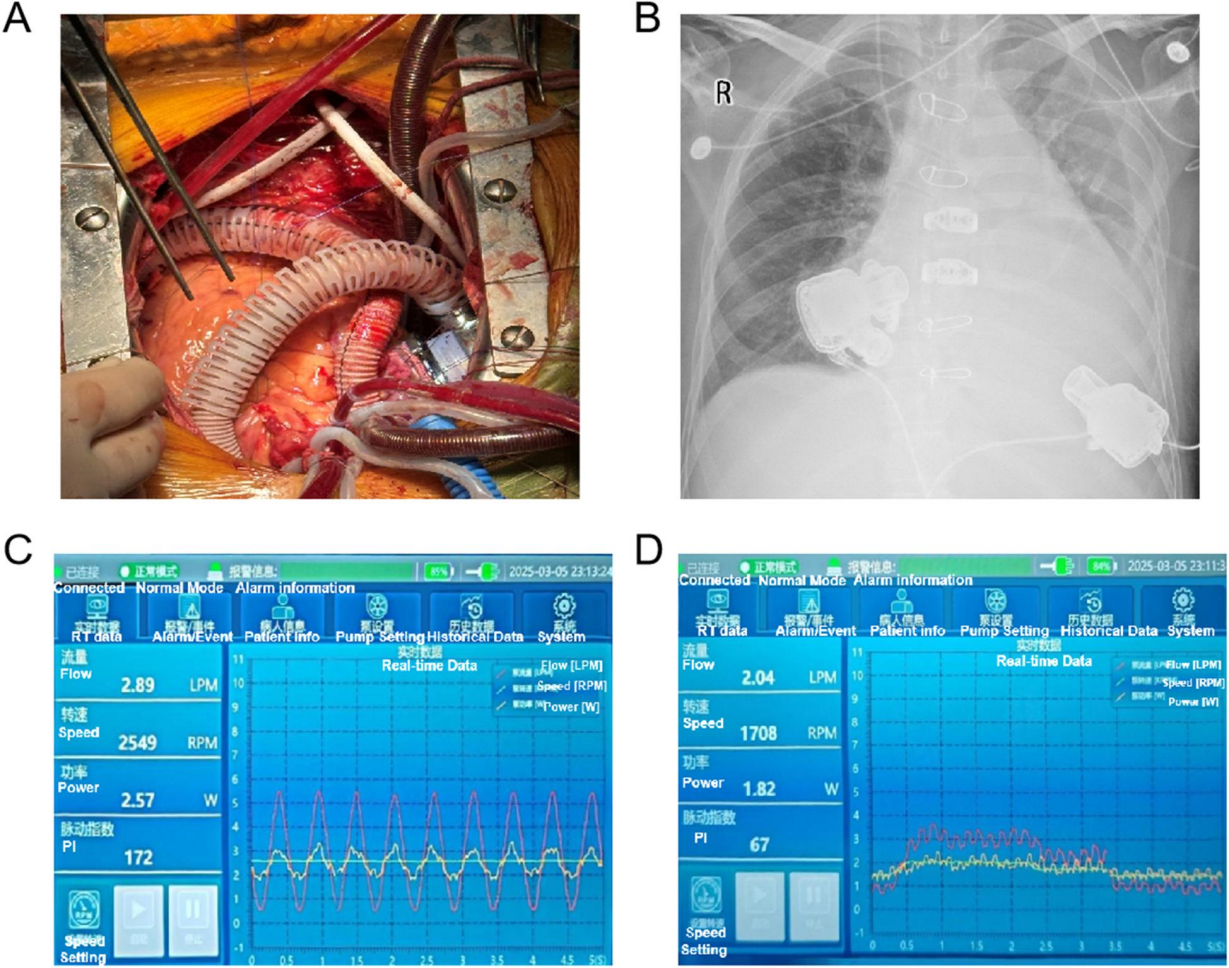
Intraoperative BiVAD implantation (A) and chest x-ray (B) in postoperative day 0 of the patient. (C) LVAD and (D) RVAD console interface displaying real-time hemodynamic parameters: flow rate, rotor speed, power, and pulsatility index (PI).
